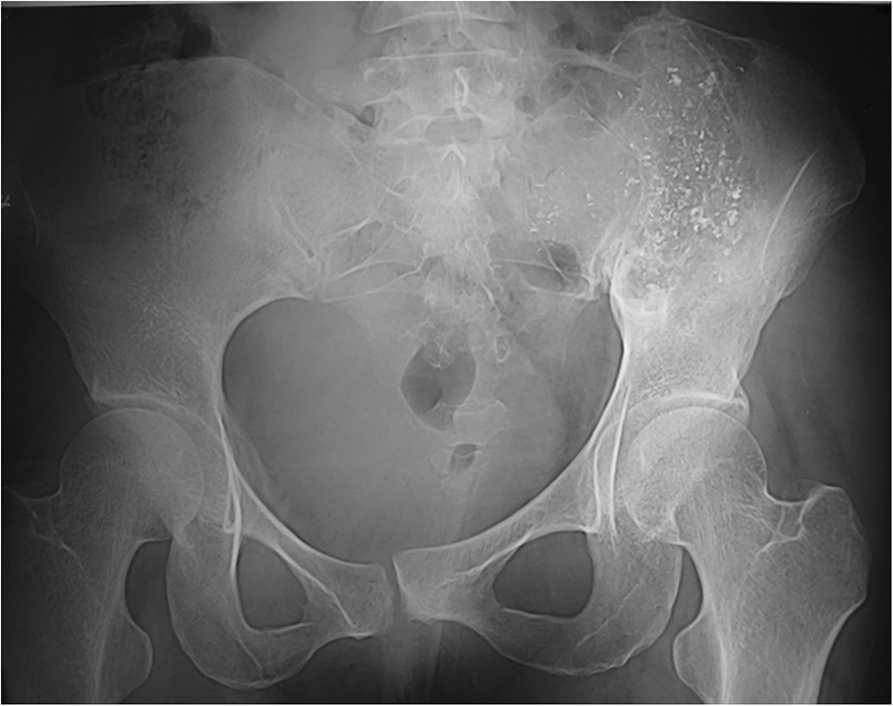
Radiography following the second embolization shows many iodized oil shadows in the osteolytic lesion.
